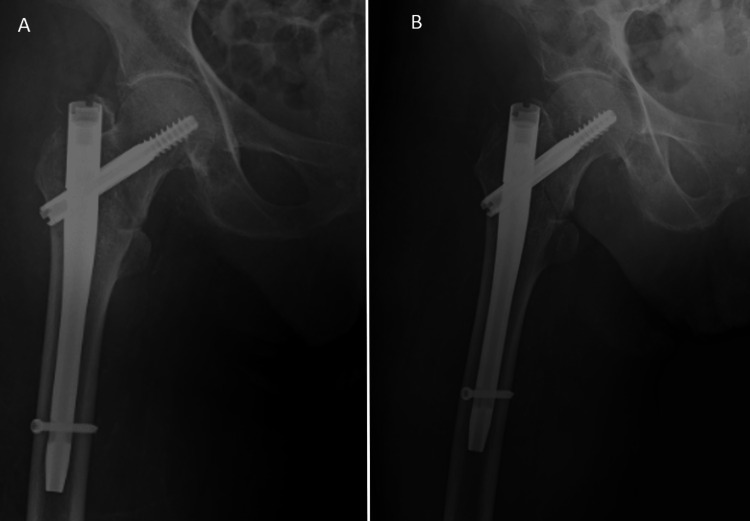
Postoperative anteroposterior (A) and oblique (B) radiographs of the right hip
